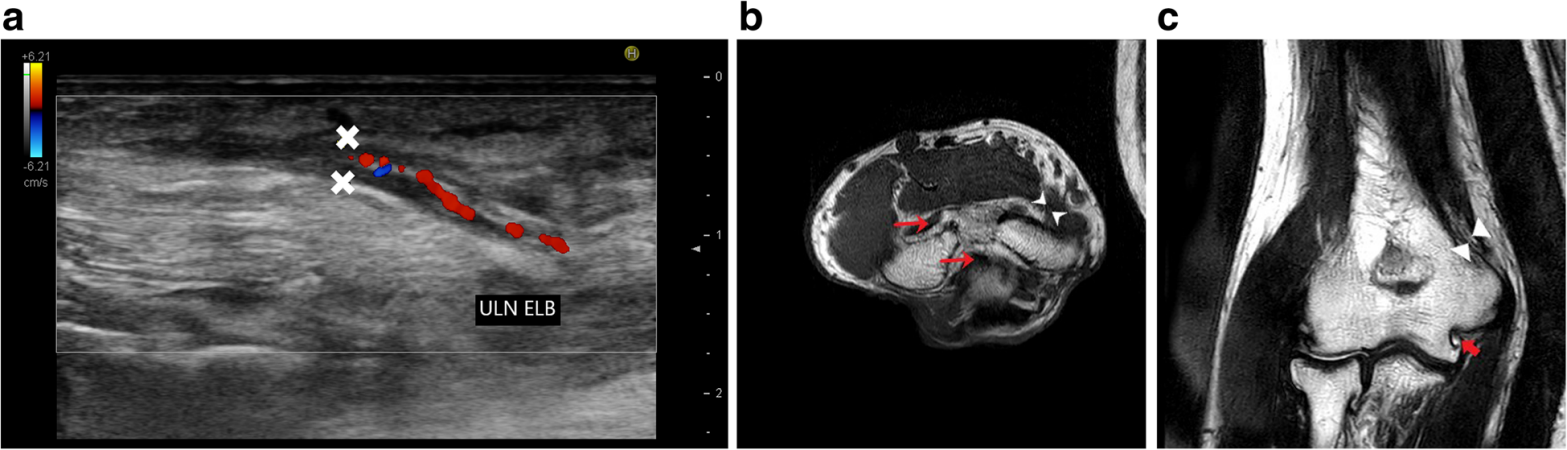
a Thickened, hypoechoic ulnar nerve with increased intra neural vascularity on colour Doppler, signifying ulnar neuritis. b Ulnar nerve (white arrow head) is hypointense and located anteromedial to the normal location anterior to the medial epicondyle (ME) in a case with anterior intramuscular transposition of ulnar nerve. There is also associated joint effusion (red arrow) which appears hypointense on this T1-weighted image. c Ulnar nerve (white arrow head) appears hyperintense and thickened on T2 coronal image lying above the medial epicondyle, passing lateral to it instead of its normal location in the cubital tunnel. Also noted are osteophytes (red arrow)
